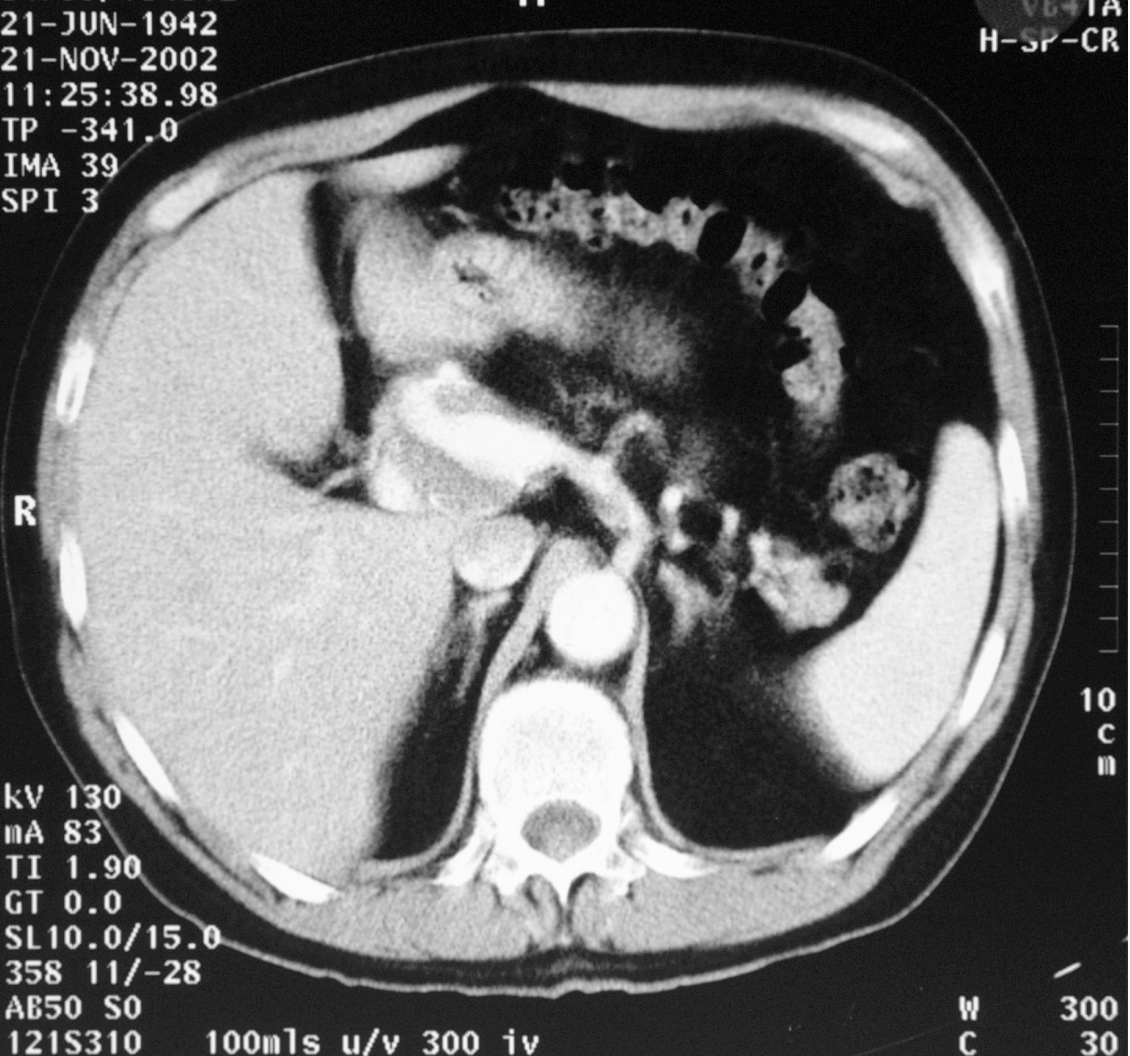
Computed tomography scan showing the hepatic artery aneurysm.
